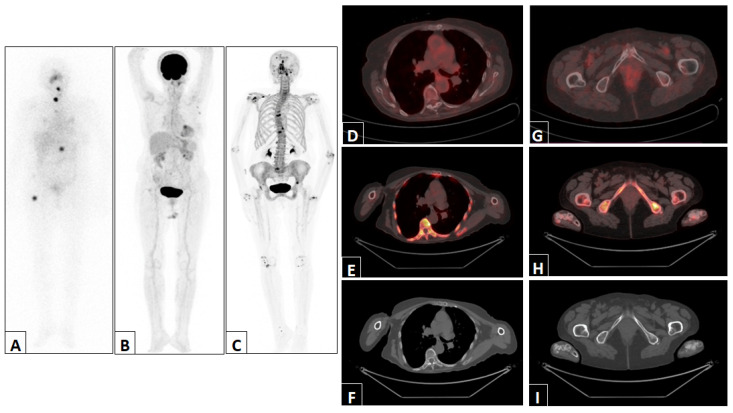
Representative example of a patient with abnormal uptake in D12 and the right femur detected via post-therapy ^131^I WBS anterior projection (A). The ^18^F-FDG PET/CT maximum intensity projection (B), ^18^F-NaF PET/CT maximum intensity projection (C) and ^18^F-FDG PET/CT, ^18^F-NaF PET/CT and CT trans-axial views of D12 (D–F) and the right femur (G–I) were normal.
